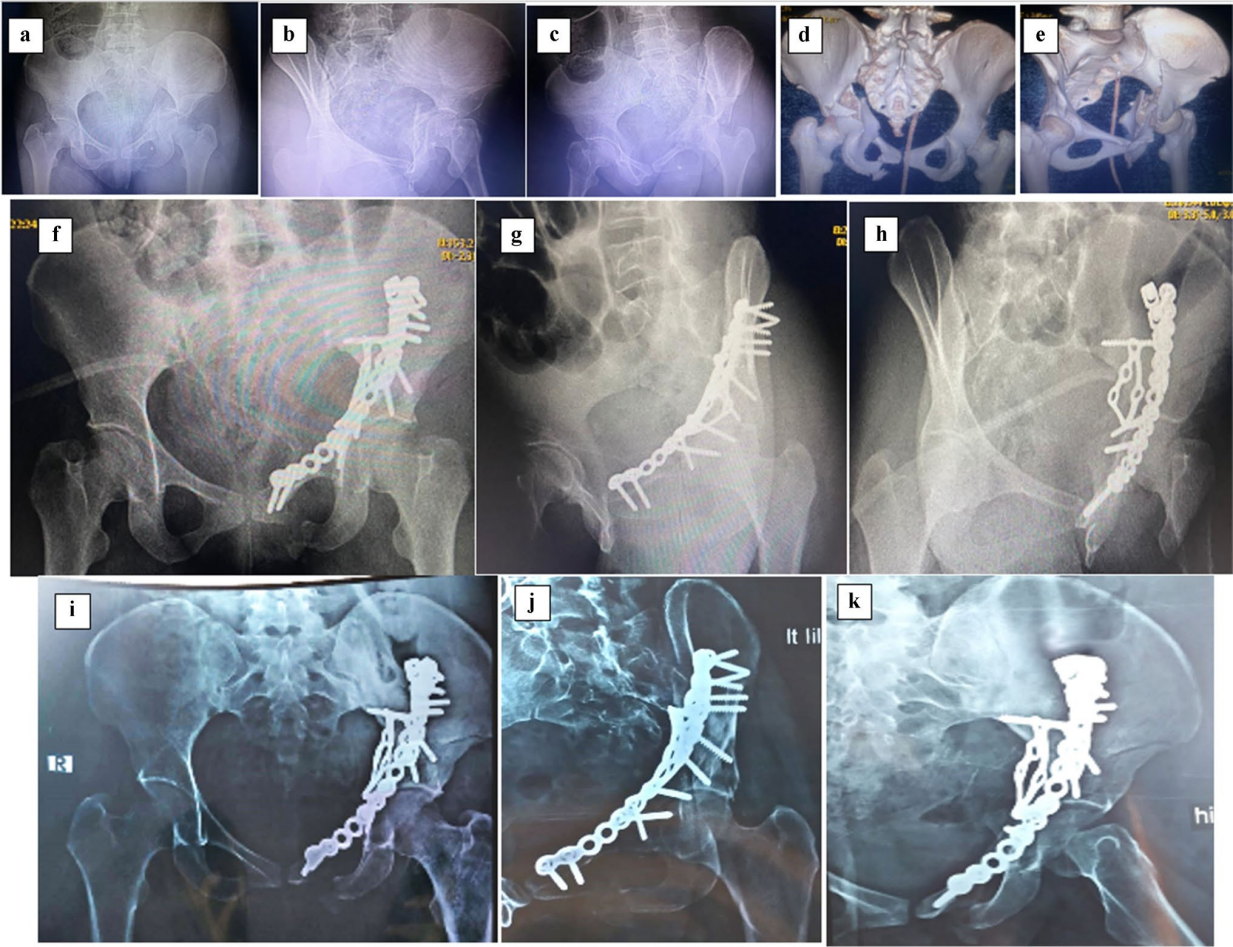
A 52-year-old female RTA. a-e preoperative radiographs and computed tomography (CT) scan cut showing Left Associated Both Column fracture acetabulum. F–h postoperative radiographs show fixation via anatomical QLP and iliac wing plating. I-l Radiographs taken 13 months postoperatively
